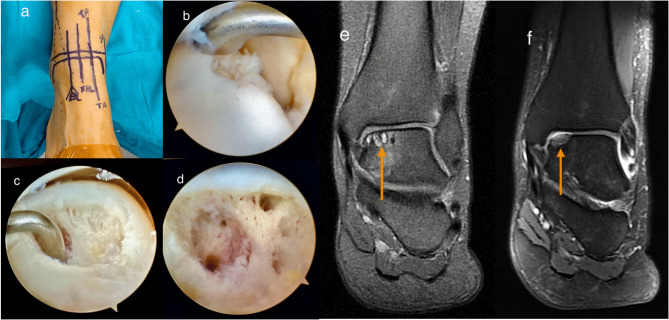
Arthroscopic debridement along with the use of the microfracture technique in non-traumatic OLTs. a Preoperative preparation for arthroscopy, b Identification of the region of the OLTs using a probe. c Post-debridement appearance of the OLTs area, d Post-microfracture appearance of the OLTs area, e Pre-operative MRI related to the lesion, f Postoperative 36-months MRI showing the defect area filled with fibrocartilaginous tissue
